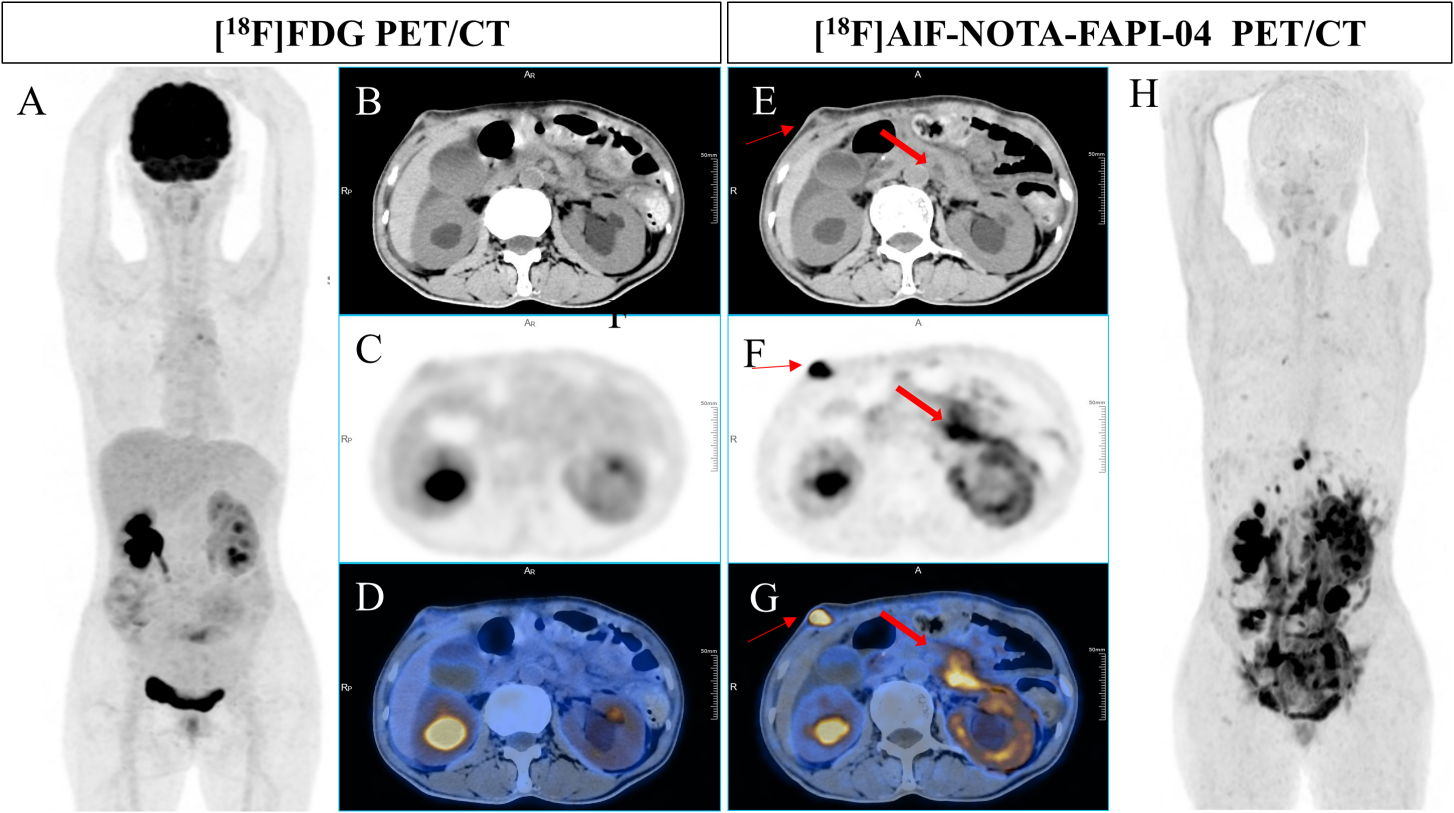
A 49-year-old man underwent radical resection for poorly differentiated adenocarcinoma of the stomach twelve months prior. Subsequent routine CT scans revealed suspected peritoneal carcinomatosis. [^18^F]FDG PET/CT imaging (A-D) demonstrated no abnormal uptake throughout the whole body. However, [^18^F]AlF-NOTA-FAPI-04 PET/CT (E-H) revealed increased tracer uptake in the thickened retroperitoneal soft tissue and a mass in the right abdominal wall (bold arrow, SUVmax of 14.5; thin arrow, SUVmax of 21.9). Pathological examination of the biopsy sample from the mass in the right abdominal wall indicated a few atypical round cells, suggesting malignancy.
